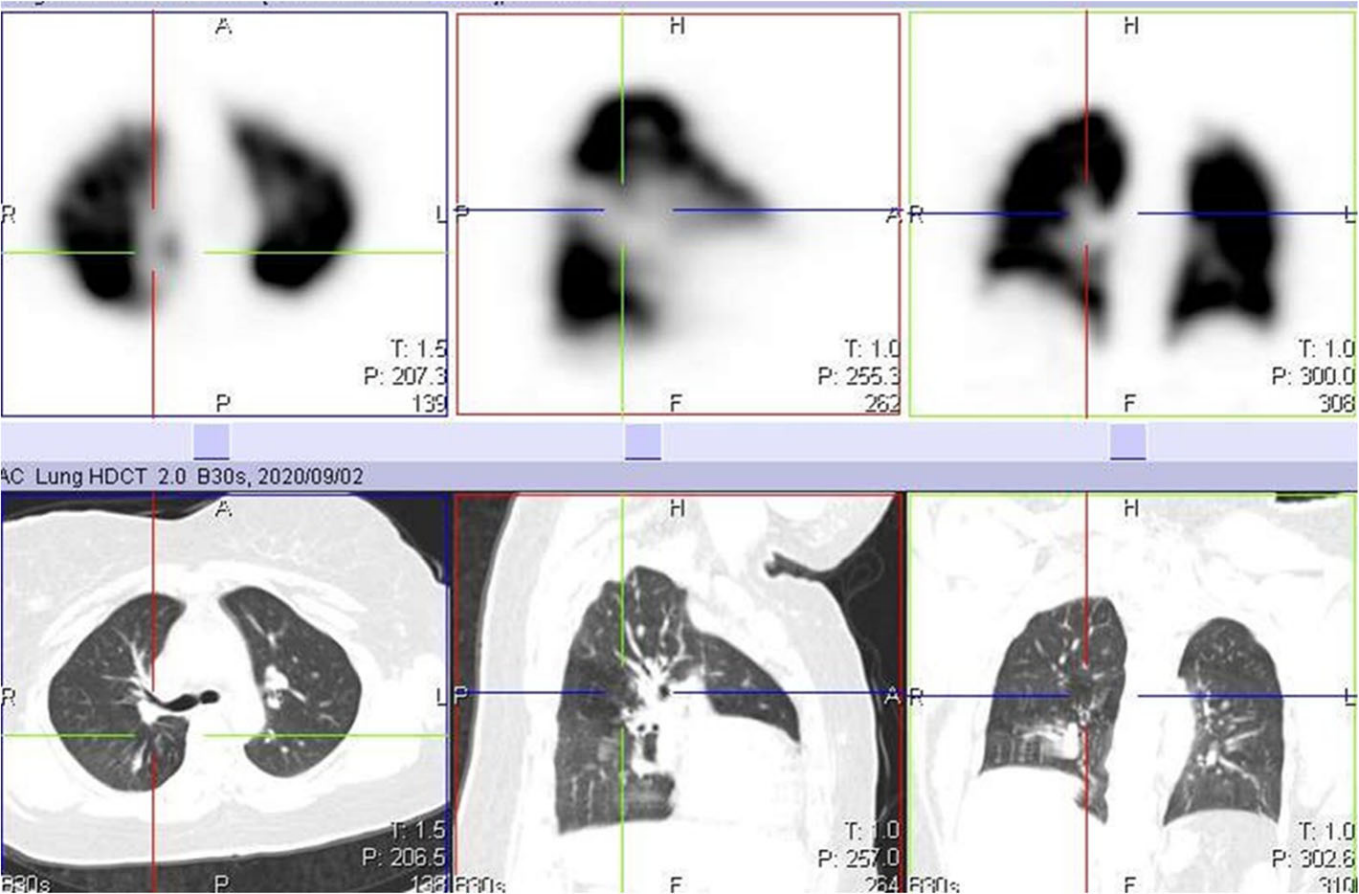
Perfusion only SPECT/CT study of a 40-year-old female, performed 12 days after testing positive for COVID-19 infection, showing a large perfusion defect in the right lung (crosshair). This was initially attributable to pulmonary embolism
